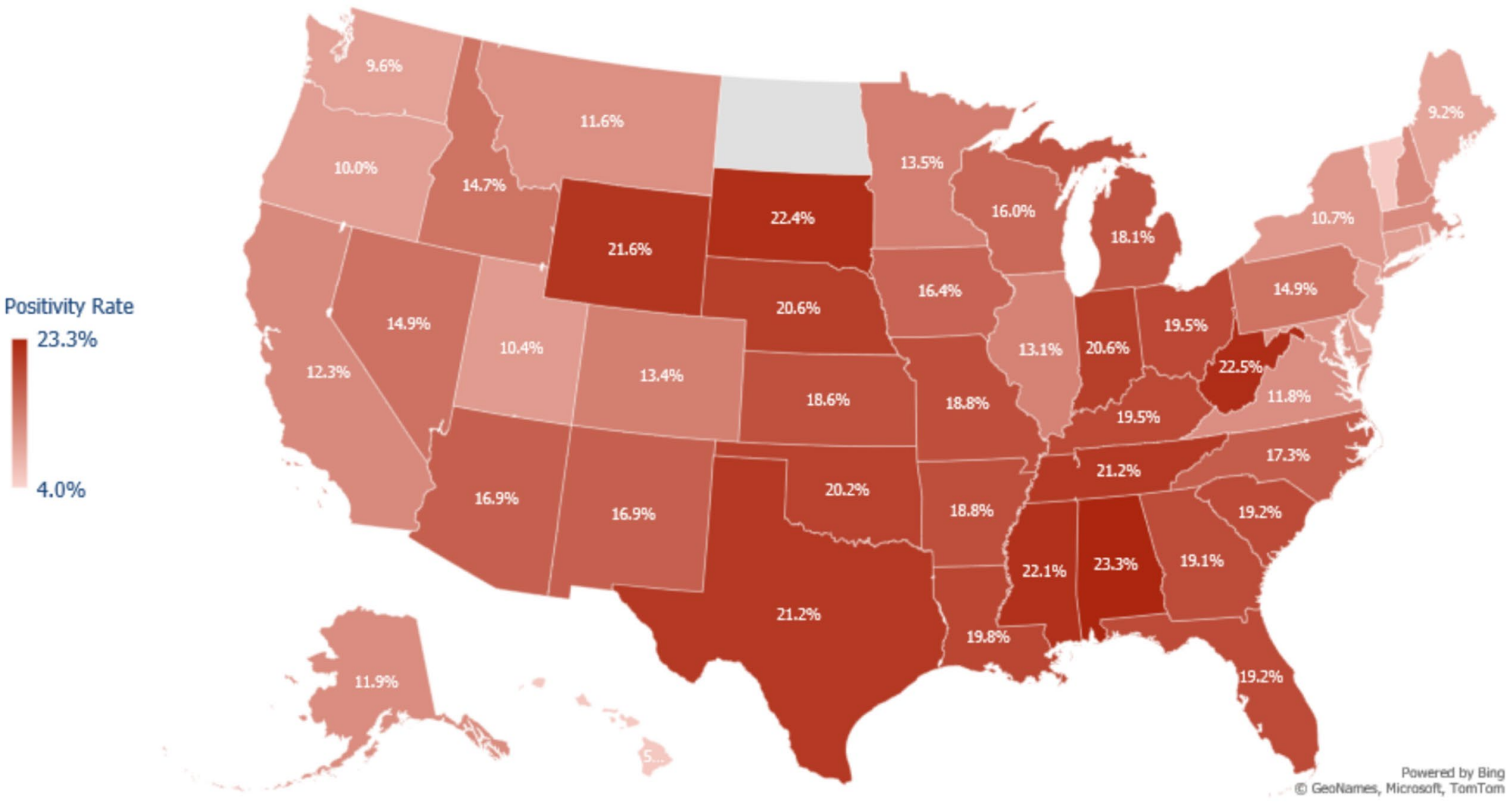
Map of COVID-19 test positivity rate in the United States among adults testing for COVID-19 at a Walgreens pharmacy or Walgreen-affiliated mass testing site between May 2021 to February 2022. Positivity was calculated as the number of positive tests for SARS-CoV-2 divided by the total number of tests administered in each state during the study period. Northeast States Positivity Rate = CT: 10.0%, DE: 9.2%, MA: 12.0%, MD: 11.4%, ME: 9.2%, NH: 12.1%, NJ: 10.1%, NY: 10.7%, PA: 14.9%, RI: 9.8%, VT: 5.3%. Walgreens does not have stores in North Dakota, so there is no positivity estimate for this state.
